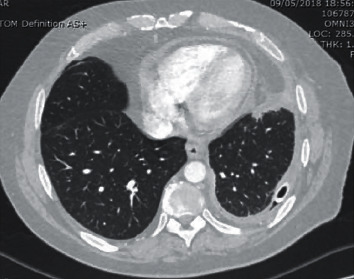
CT after readmission and redrainage.
